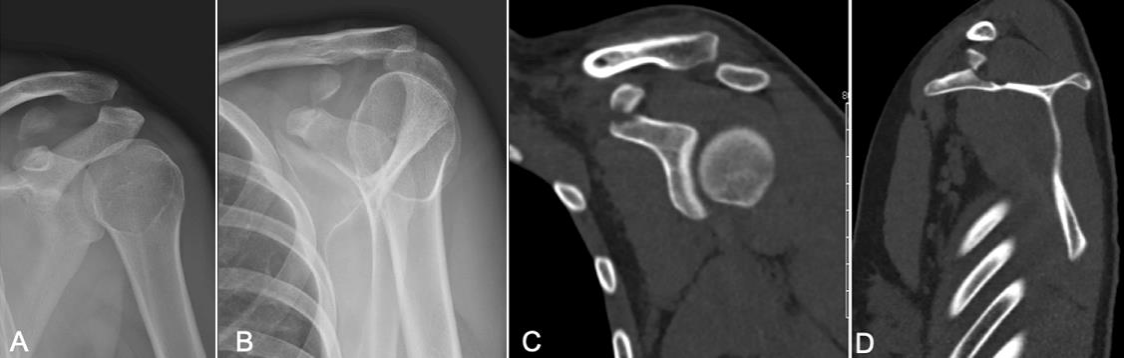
preoperative imaging, A) AP X-rays; B) lateral X-rays; C) CT-scan coronal view; D) and sagittal view
